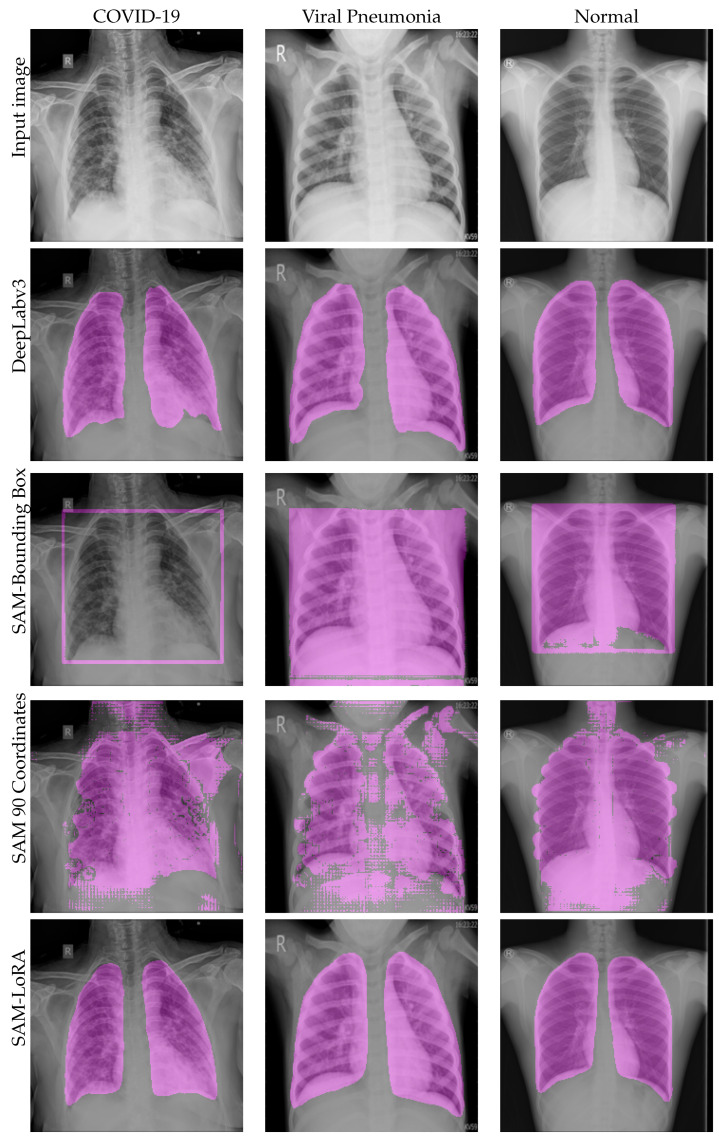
Avisualization of the performance of different models on three categories of chest X-ray images. The masks are overlaid on the original image in purple.
